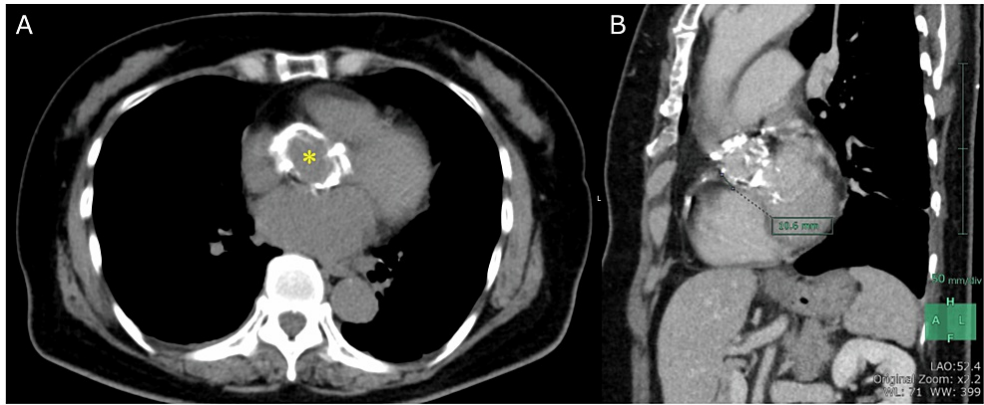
Preoperative chest computed tomography Computed tomography showed severe calcification at the level of the sinus of Valsalva (asterisk) (A). The distance between the right coronary artery ostium and the aortic annulus was 1.06 cm.
